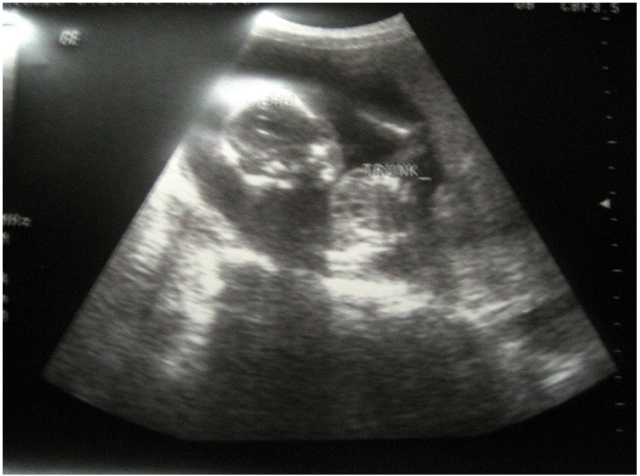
Ultrasound abdomen with live 17-week intrauterine fetus and large amount of free intraperitoneal fluid
